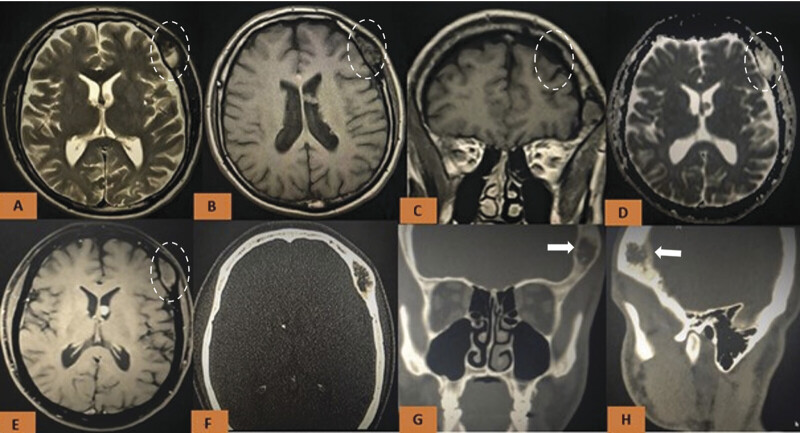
Left frontal epidermoid cyst. A hyperintense, intradiploic cystic lesion (41 × 26 × 22 mm) on axial T2-weighted MR images (
